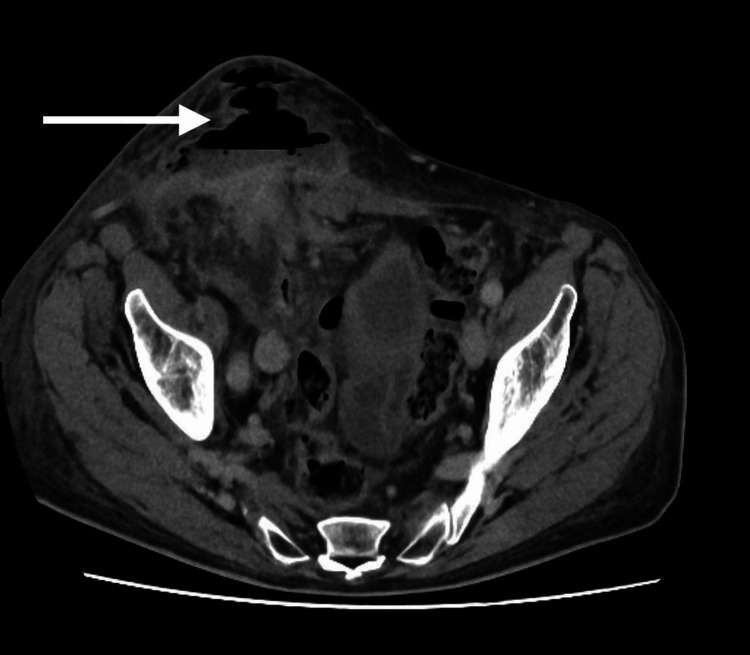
CTAP axial plane - 1 Collection suggestive of colon perforation CTAP: computed tomography of the abdomen and pelvis
